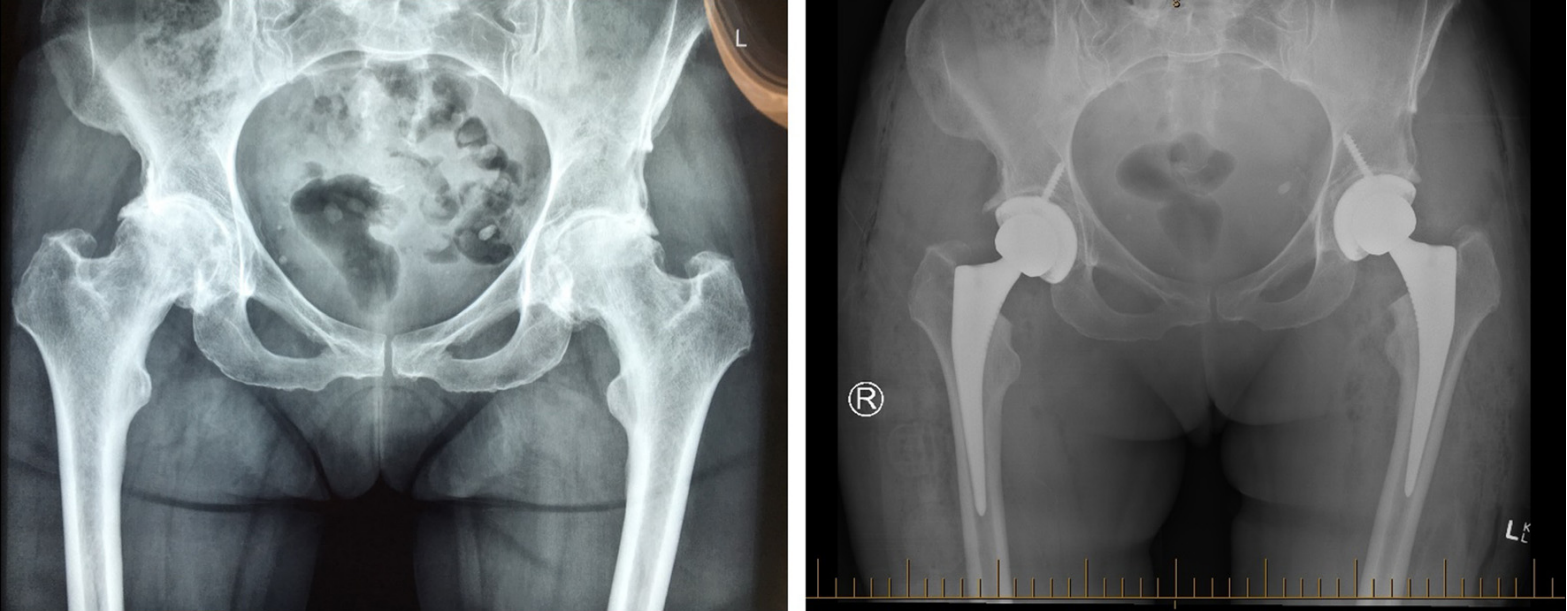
Pre-operative AP X-Ray pelvis with both hip joints (Case 2) showing grade IV OA both hip joints and post-operative X-ray after bilateral uncemented bikini hip replacement showing well aligned acetabular and femoral prosthesis in situ (Case 2).
